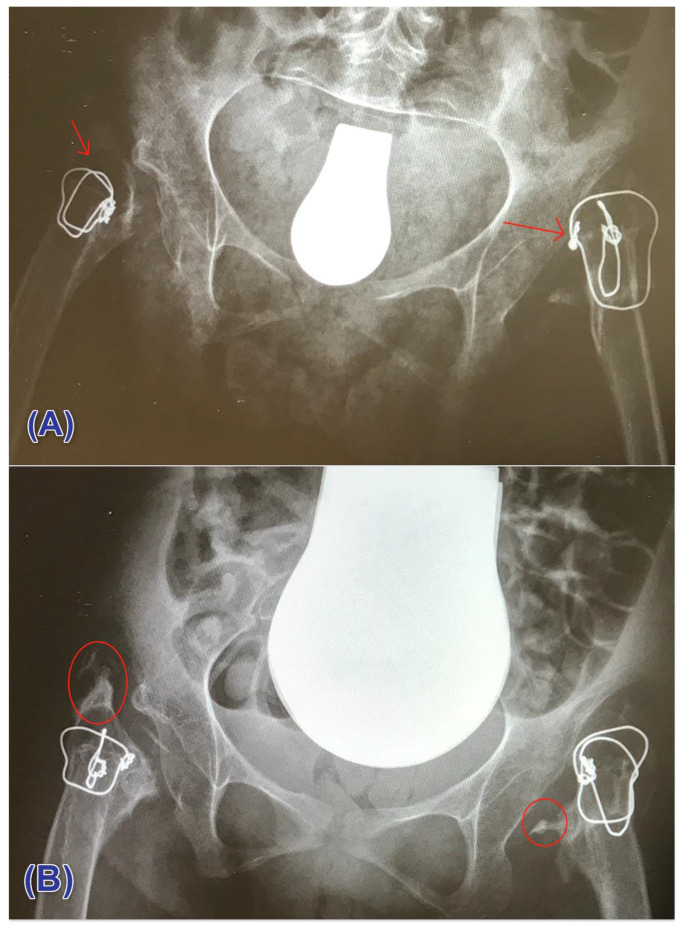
Postoperative radiograph showing (A) no ossification (at the site of the arrow) (B) heterotopic ossification (encircled).
